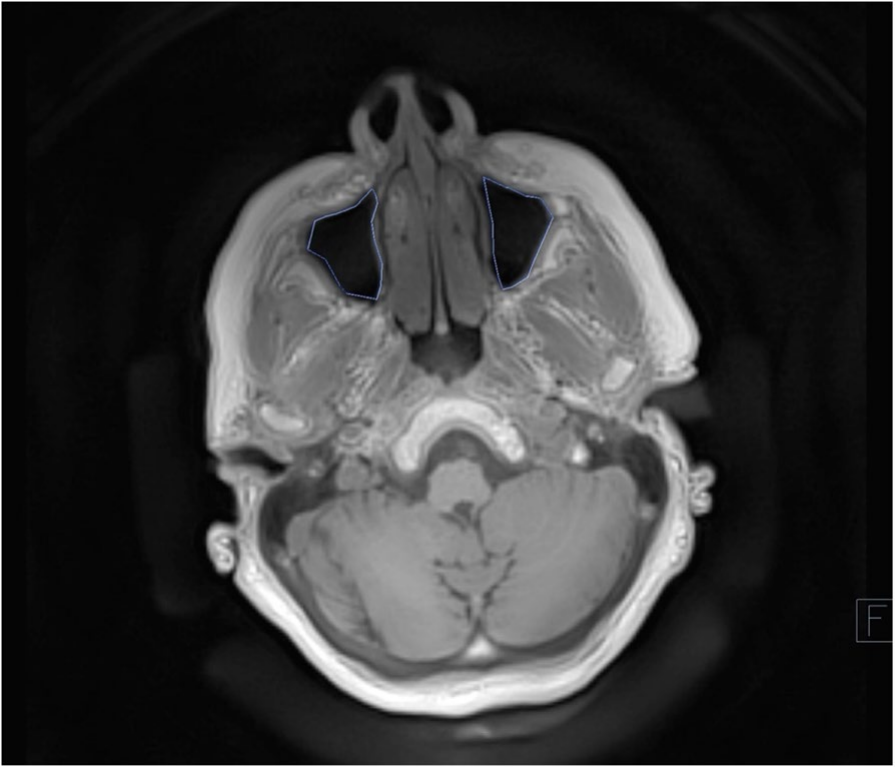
Preoperative MRI scan: marking of the outline of the maxillary sinus, transversal layer, T1 weighted petra sequence
